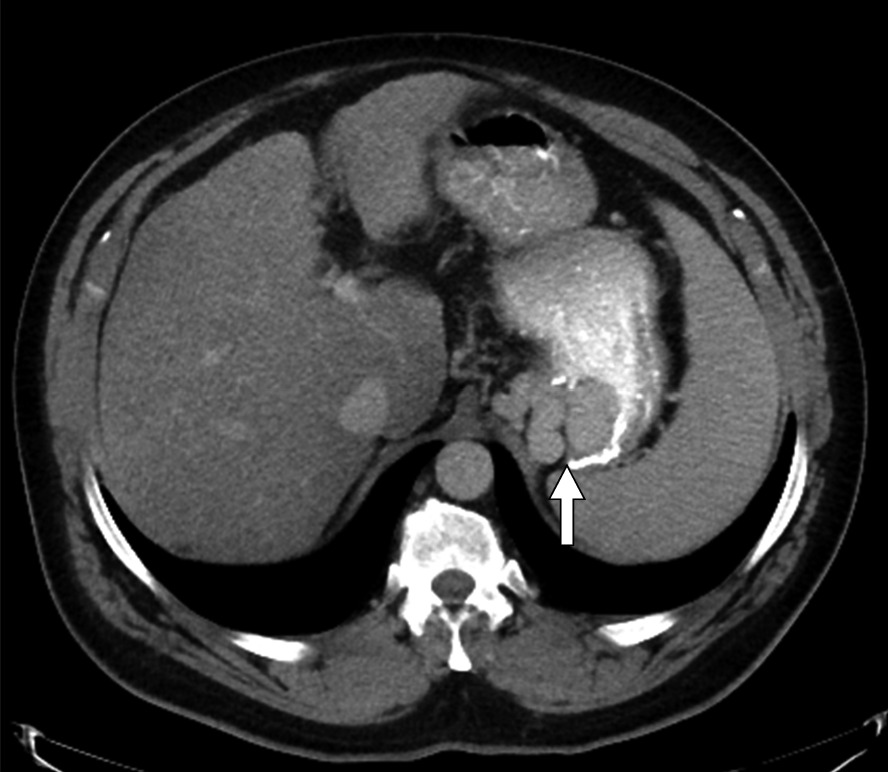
Axial contrast enhanced CT image showing dilated gastric varices (arrow)
